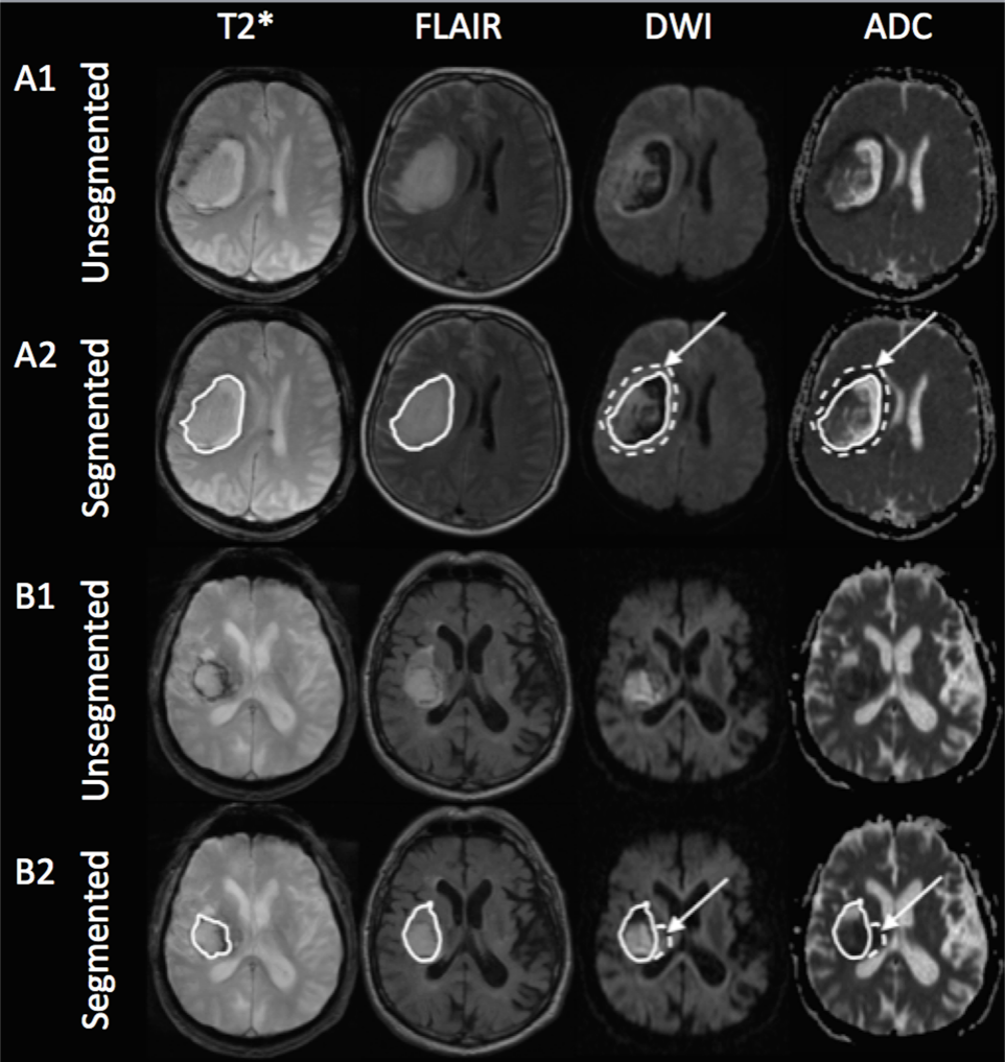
Two examples of unsegmented (A1 and B1) and segmented (A2 and B2) hyperacute hypertensive right-sided intraparenchymal hemorrhages (IPH) on co-registered axial T2*, fluid-attenuated inversion recovery (FLAIR), diffusion-weighted imaging (DWI), and apparent diffusion coefficient (ADC) images. Example A shows the MRI of a 51 year old male patient 1.5 hours after symptom onset and example B that of a 82 year old male patient in a 3-hour window. The solid line on T2* (A2 and B2) shows the unadjusted IPH ROI and that on FLAIR, DWI, and ADC the adjusted whole lesion ROI (IPH+PHE, IPH ROI is not shown here for the sake of clarity). The dotted line with arrows on DWI and ADC represent the PDR ROI.
